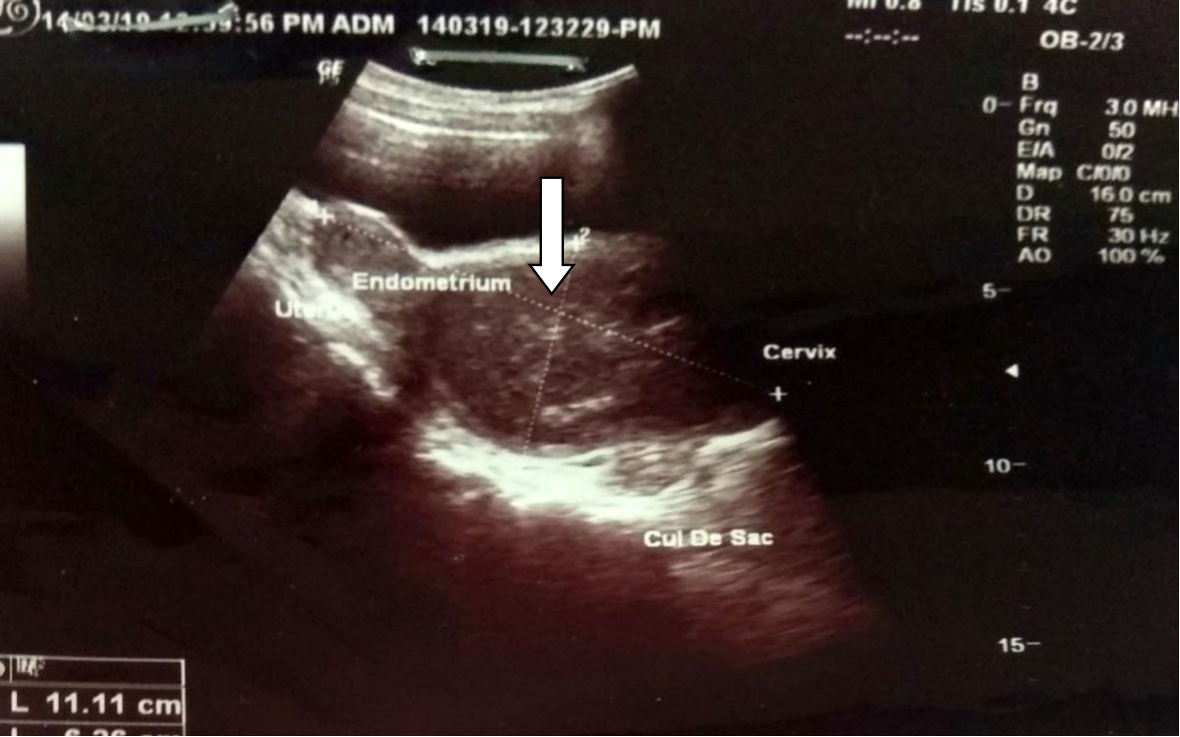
Ultrasonogram of lower abdomen showing endometrial cavity (*arrow*) filled with large, thick collection that extends up to the cervix
